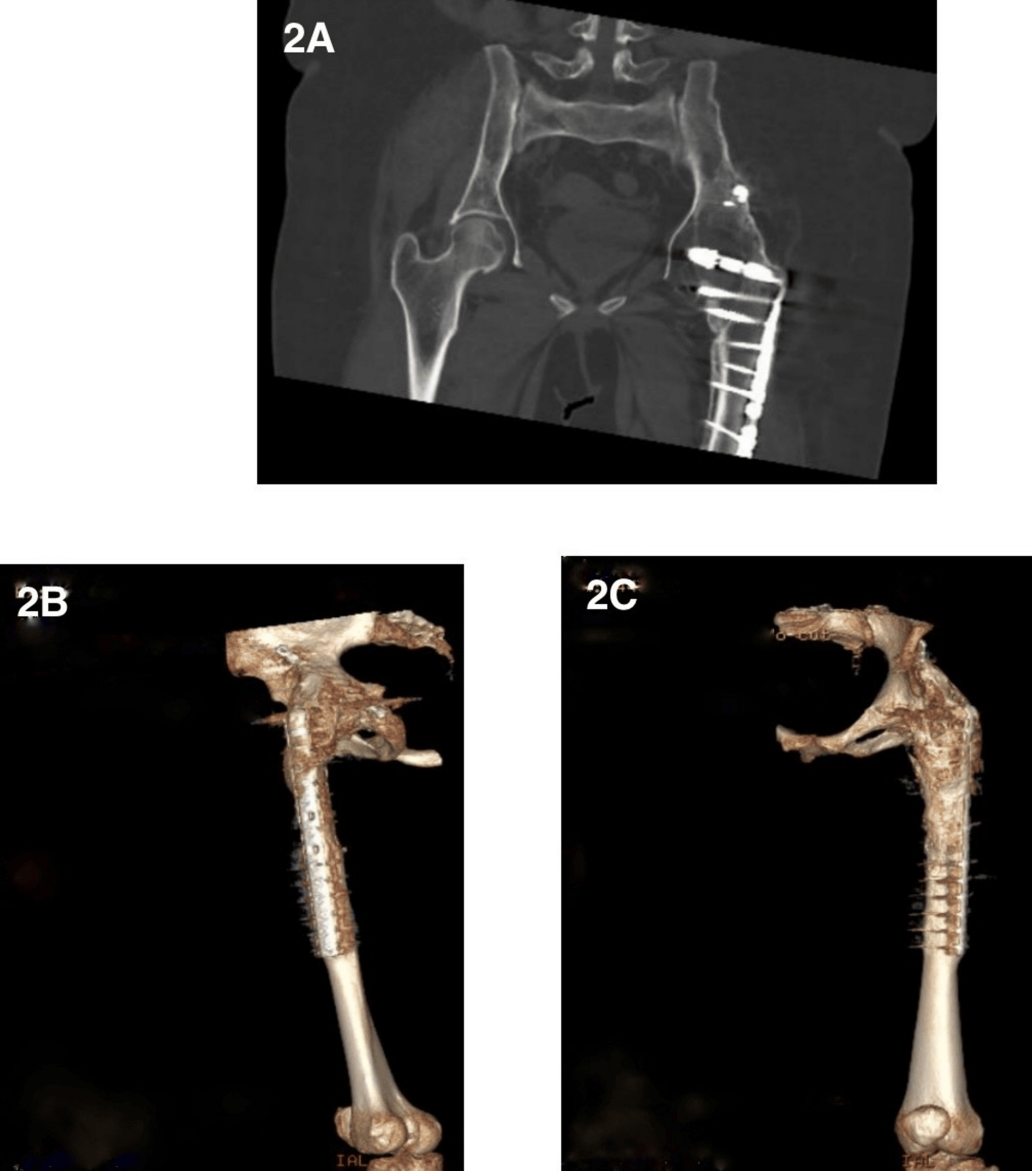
CT of the left hip joint Preoperative CT scan showing the core pelvis (A) and a 3D view of the left femur (B/C), confirming findings of the plain radiographs - hip arthrodesis and open reduction and internal fixation of the left proximal femur CT: computed tomography
